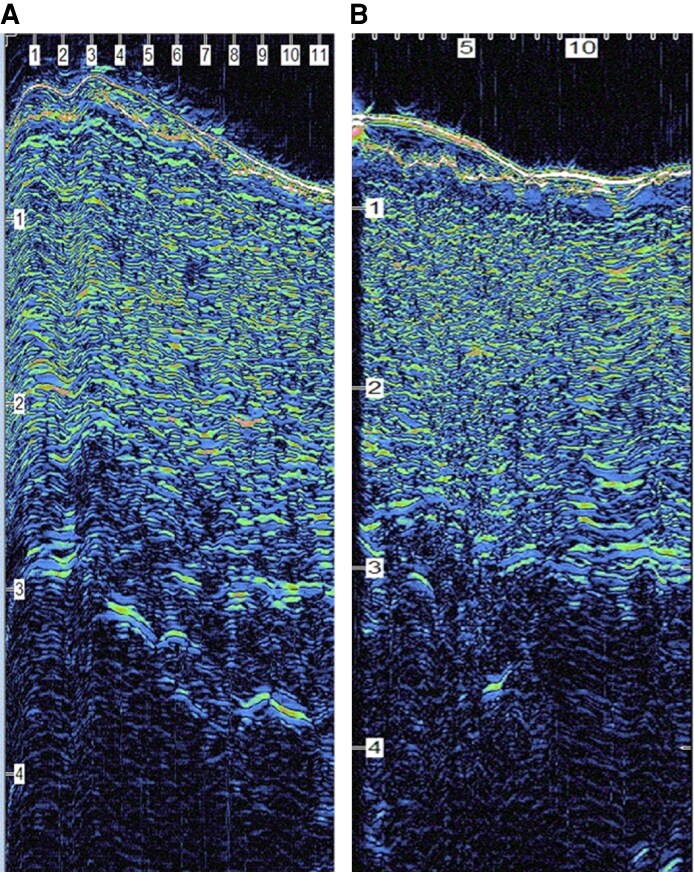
Ultrasound image of an abdominoplasty 6 weeks postsurgery (A) with bland moisturizer and (B) with R&R—showing decreased fibrous banding in B (less debris within subcutaneous tissue and better separation between dermis and subcutaneous tissue). Subtle but noticeable changes typical of ultrasound results observed.
